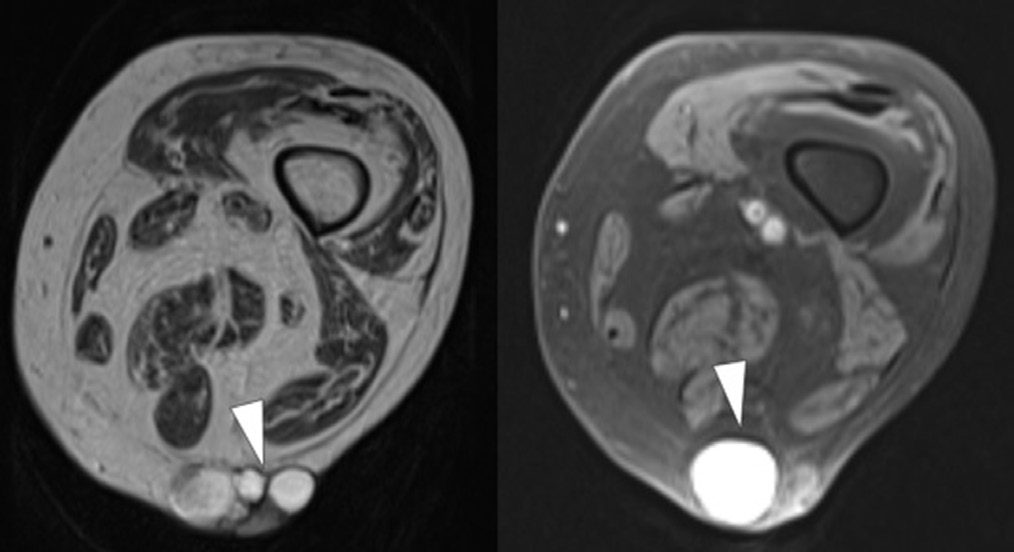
Axial MRI of the skin mass showing the cystic components with heterogeneous signal on *T*
